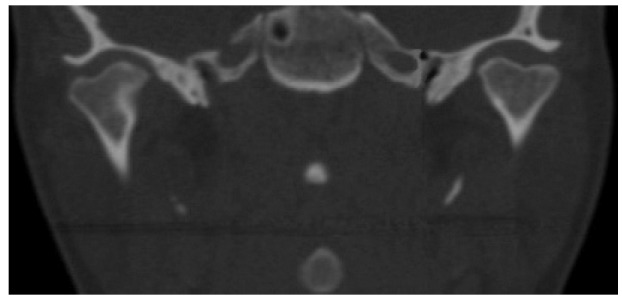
Coronal CT scan of the patient (Patient 3) revealed a bilateral bifid mandibular condyle.
